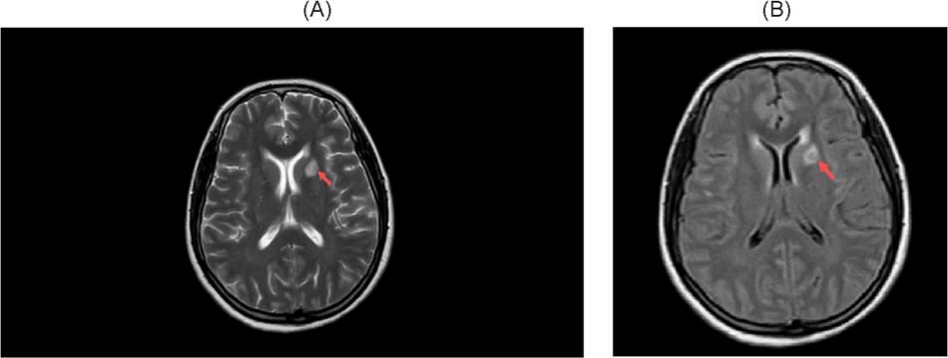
MRI of head. (a) T2-weighted view. (b) FLAIR view. Red arrows showing ring-like lesion in the left lentiform nucleus.
